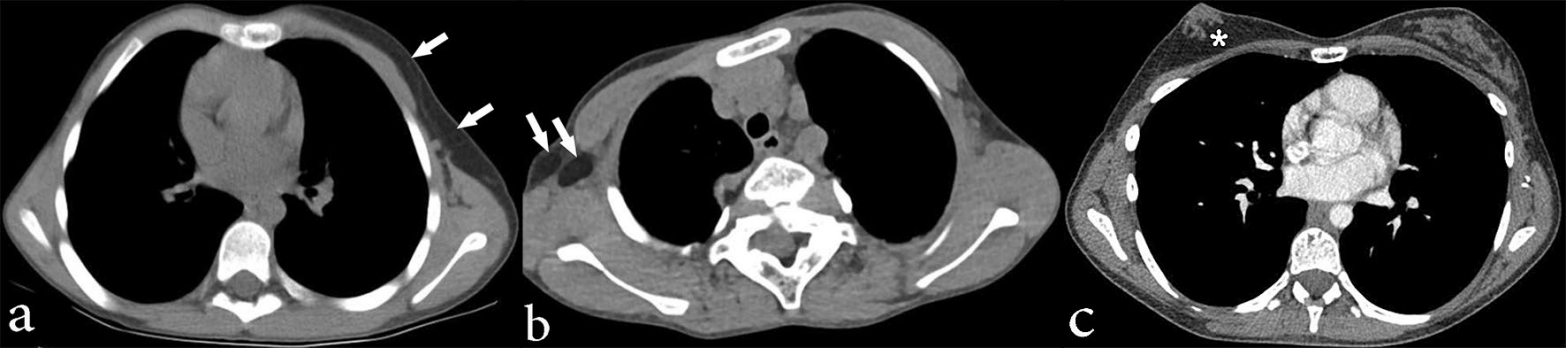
Axial chest CT images in soft tissue windows depict abnormal adipose overgrowth in individuals with Proteus Syndrome. Axial CT images show (a) asymmetric fat overgrowth in the left chest wall (arrows) in a 20-year-old male; (b) well-circumscribed areas of fat likely represent lipomas within the right axilla (arrows) in a 9-year-old male; (c) asymmetric breast tissue in a 22-year-old female. There is a paucity of breast tissue in the right breast with an increased amount of adipose tissue (asterisk).
